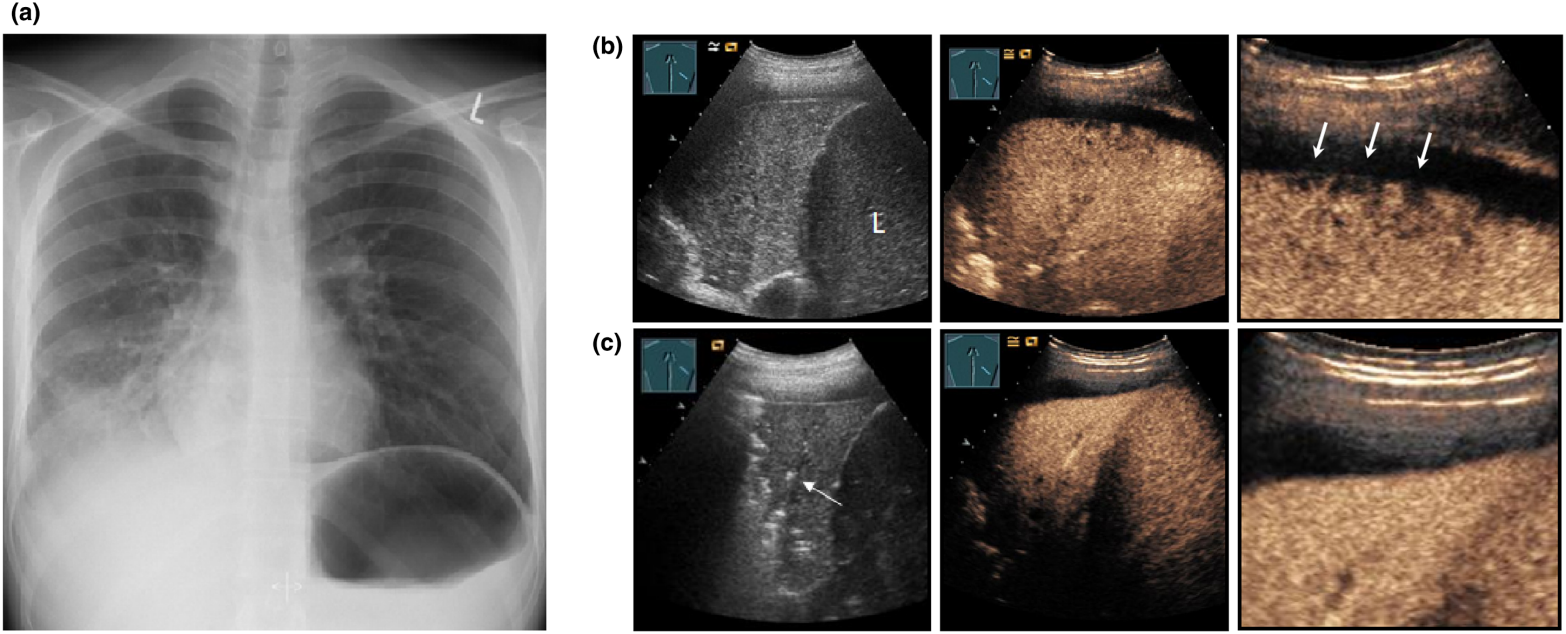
A 21‐year‐old patient with fever, loss of appetite, and weakness presented to the emergency department. (a) Presentation of a right‐sided consolidation on chest X‐ray (courtesy of Prof. Dr. Andreas H. Mahnken, Department of Radiology, University Hospital Marburg); Series (b) B‐mode ultrasound shows an inhomogeneous, hypoechoic consolidation (L = liver); on contrast‐enhanced ultrasound, after 2 minutes, the consolidation reveals a peripheral, moth‐like pleural area without contrast enhancement in overview and magnification (arrows); Series (c) after 2 days, there is regression of the consolidation with a mild air bronchogram (arrow); furthermore, a complete reperfusion of the peripheral non‐perfused areas can be observed, indicating a community‐acquired pneumonia with primarily disturbed, peripheral, pulmonary arterial and bronchial arterial perfusion. Final diagnosis was pneumonia due to *Mycoplasma pneumoniae*.
